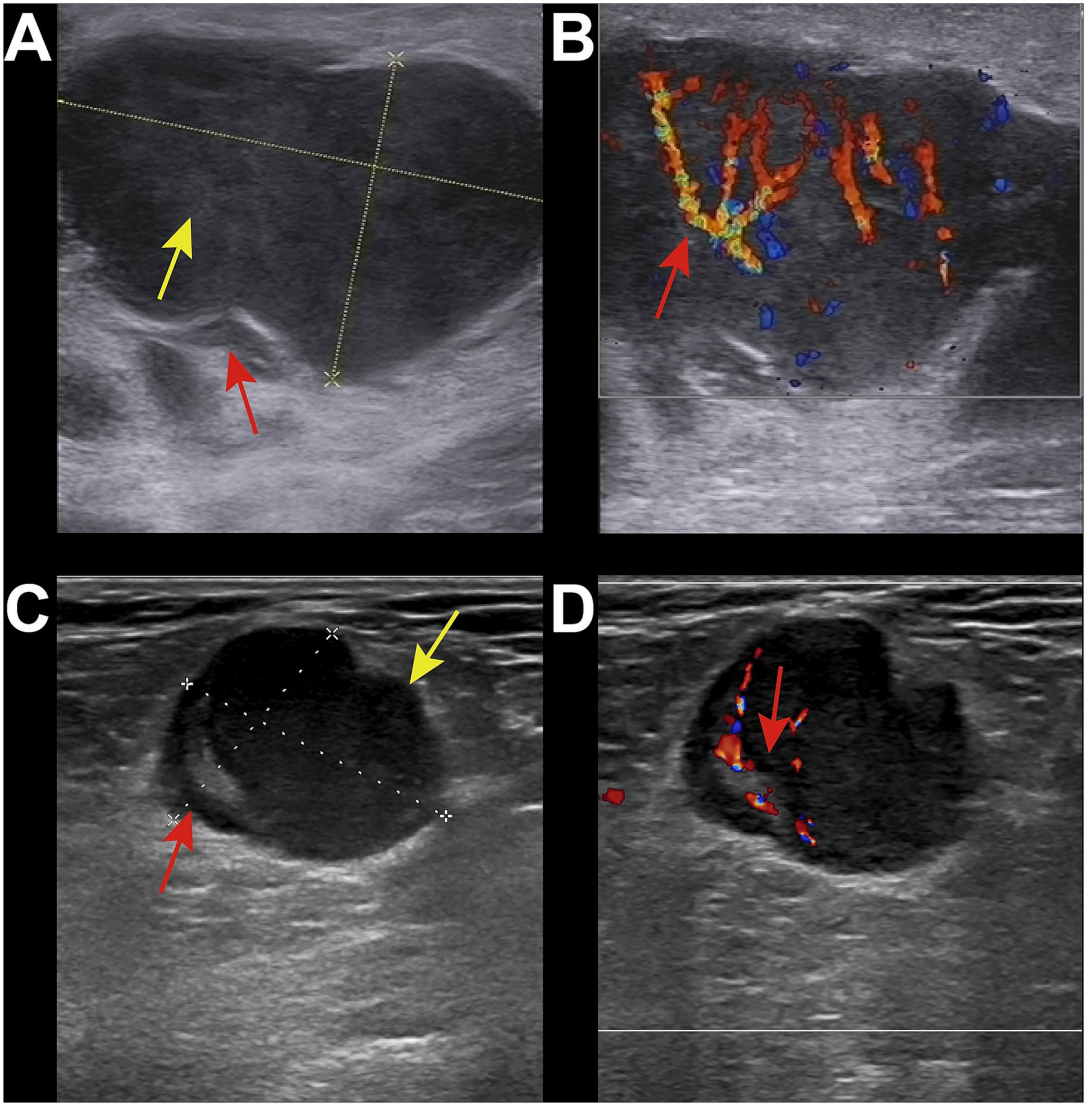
Ultrasound images of malignant group. (A) 34-year-old male, diagnosed with HIV infection for 1 year. Right inguinal lymph node enlargement was with local capsule indentation and irregular shape (red arrow). The internal echogenicity showed a reticular pattern (yellow arrow), with the absence of the lymph node hilum. (B) CDFI showed abundant blood flow signals (red arrow). The pathological diagnosis was non-Hodgkin lymphoma-diffuse large B-cell lymphoma. (C) 50-year-old male, diagnosed with HIV infection for 5 years. Enlarged lymph node was in the right inguinal region with irregular shape and significant asymmetric cortical thickening (red arrow). The lymph node hilum was present but compressed and eccentric (green arrow). The internal echogenicity was relatively uniform. (D) CDFI showed abundant hilar blood flow signals (red arrow). The pathological diagnosis was metastatic squamous cell carcinoma.
